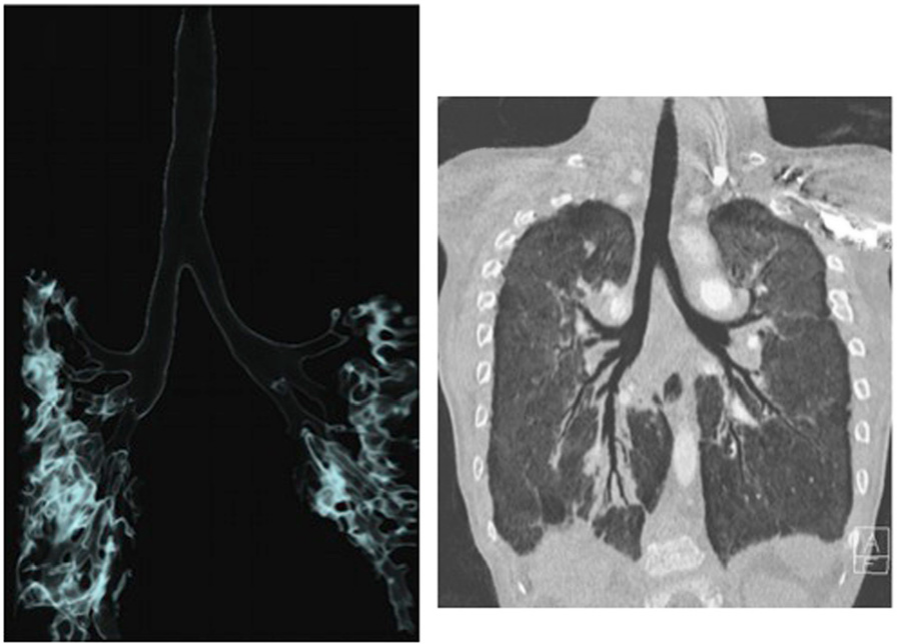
Computed tomography angiography shows long main bronchus, two hyparterial bronchi and bilobed lungs (consistent with left bronchial isomerism), several consolidative opacities, widespread peripheral cylindrical bronchiectasies, bronchial dilatation and bronchial wall thickening.
